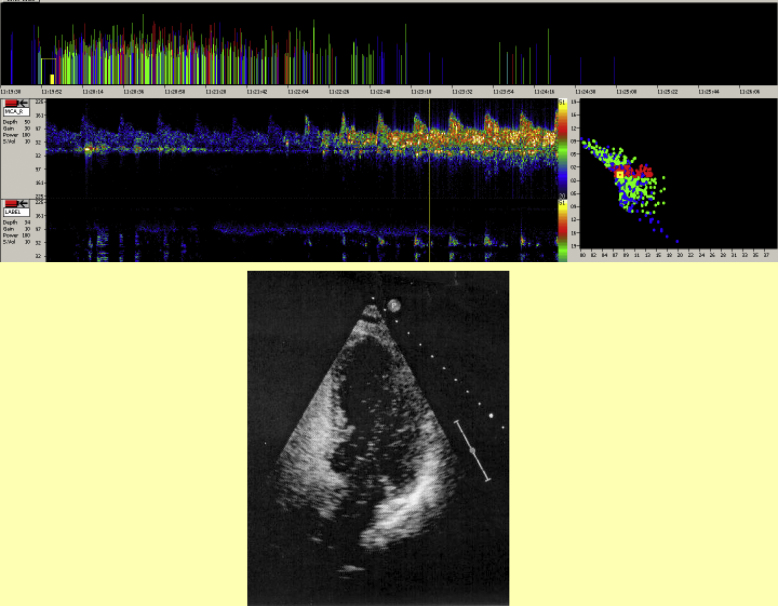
Excluding a right to left shunt. Top: Positive Patent Foramen Ovale study using a DWL EmboDop transcranial Doppler system that uses multifrequency and multiple depths simultaneously. The upper window shows the output from a 2MHz probe at 55mm depth and the lower window is 2MHz at 38mm depth (the reference gate) which in this case demonstrates that the embolic shower that is seen at 55mm is not an artefact as it is not shown simultaneously at the reference gate. Bottom: Echocardiogram during bubble contrast showing passage of bubbles from the right to the left atrium.
